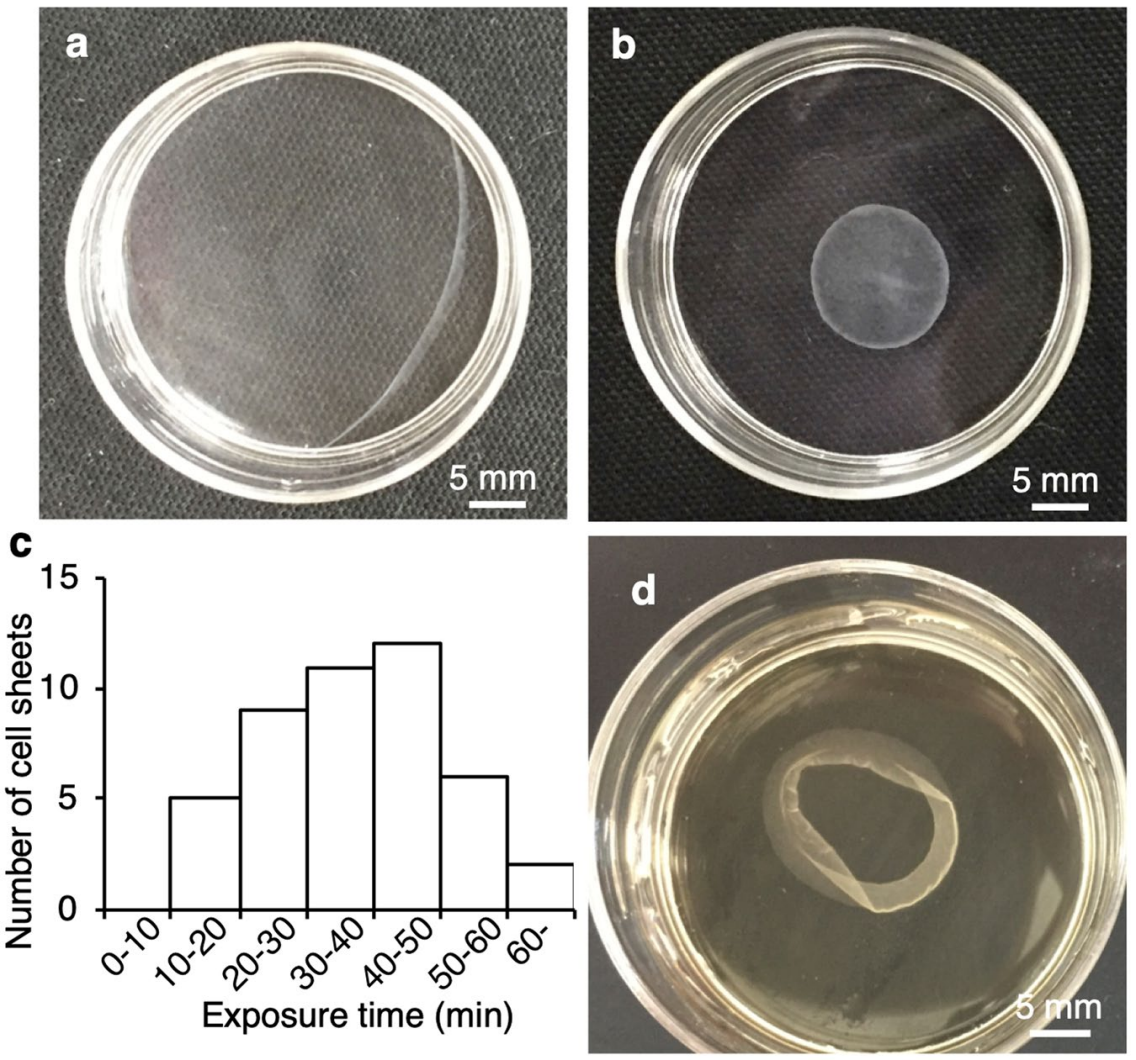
Cell sheet detachment by ultrasonic vibration. Cell sheets after ultrasonic exposure for 1 h at input voltages of (a) 12.5 V or (b) 25 V. (c) Number of cell sheets successfully detached with the corresponding duration of ultrasonic exposure and input voltage of 25 V. The total number of trials was 45. “Number of cell sheets” indicates how many cell sheets were detached at each 10 min of ultrasonic exposure. (d) Appearance of cell sheets detached at an input voltage of 25 V.
